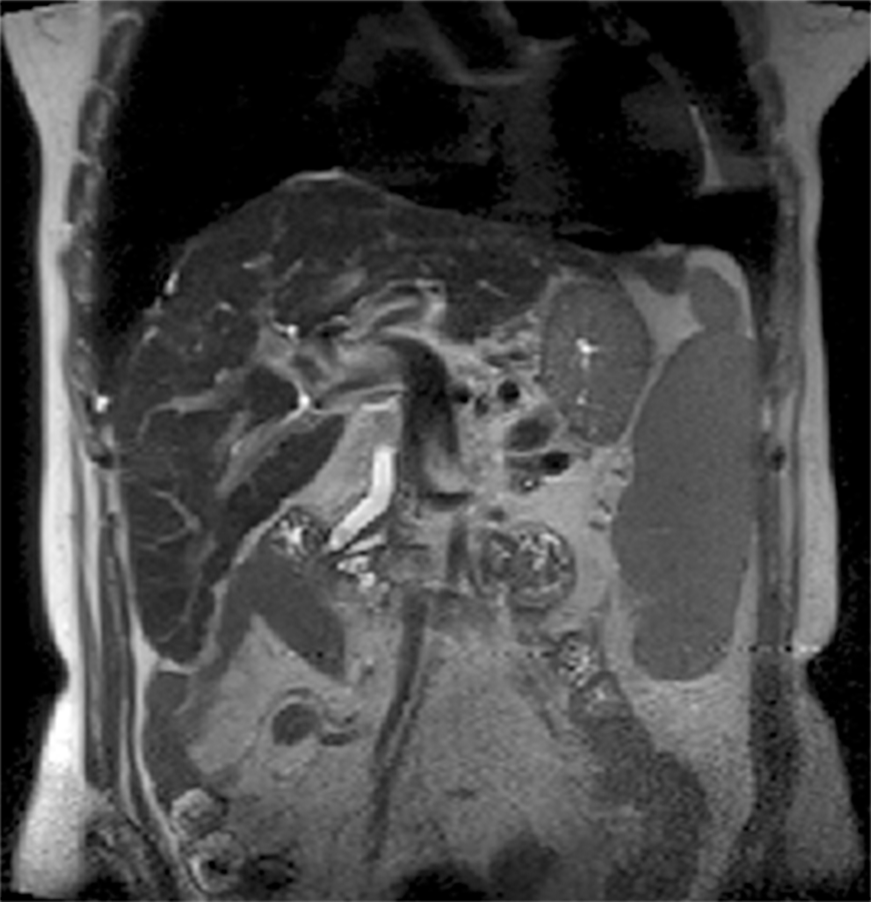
Abdominal MRI (T2-weighted sequence, coronal slice), showing a lobulated cyst within the uncinate process of the pancreas, with slight parietal thickening. Same patient as Fig. 4.
